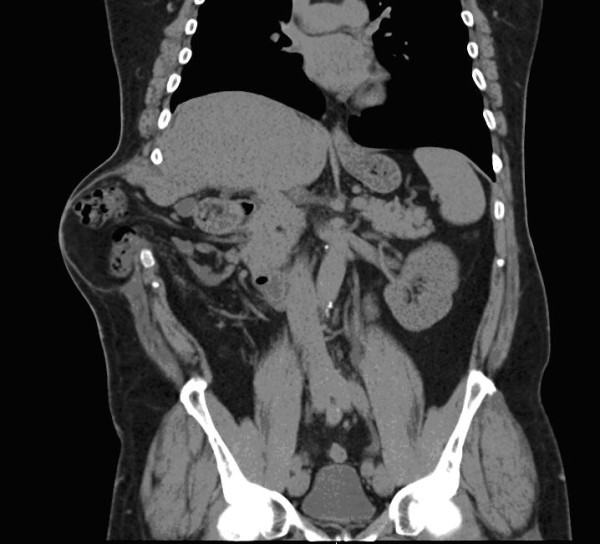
Coronal CT view: Hepatic colonic flexure and some liver tissue are included in the sac of TTIH.
